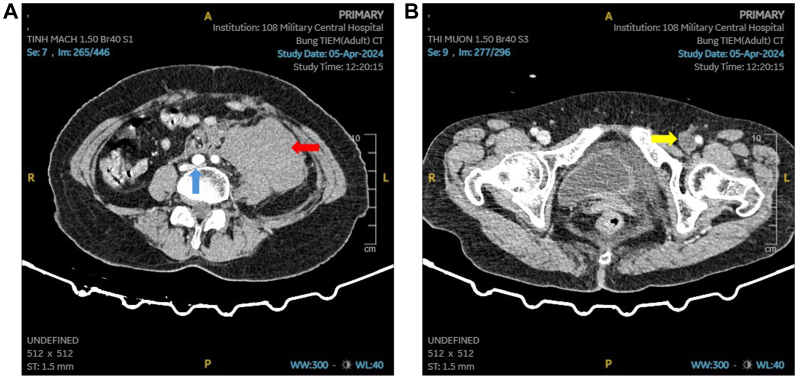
(A) A transverse computed tomography (CT) angiogram of the abdomen and pelvis demonstrated a large left-sided retroperitoneal hematoma consistent with extensive hemorrhage, without evidence of active bleeding (*red arrow*). The left common iliac vein is compressed between the right common iliac artery and the lumbar spine, consistent with May-Thurner syndrome (MTS) (*blue arrow*). (B) Complete occlusion of the external iliac veins (*yellow arrow*).
